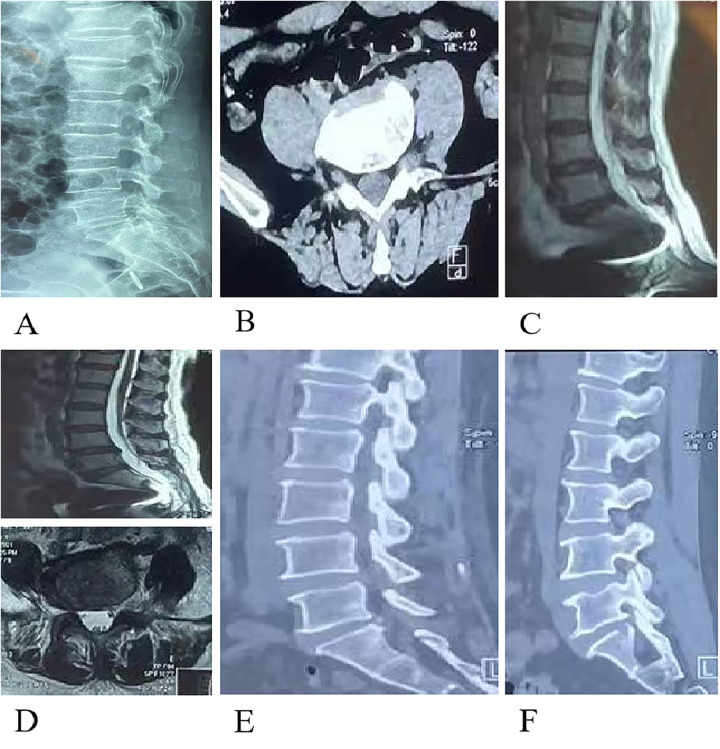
Preoperative and postoperative imaging in a patient with lumbar disc herniation treated with UBE. (A) Preoperative lateral lumbar radiograph indicates lumbar disc herniation at the L5-S1 level. (B,C) Preoperative lumbar CT and MRI show distal disc herniation at the L5-S1 level. (D–F) Lumbar CT and MRI performed 3 days postoperatively reveal partial absence of the lamina, with no significant abnormalities detected.
